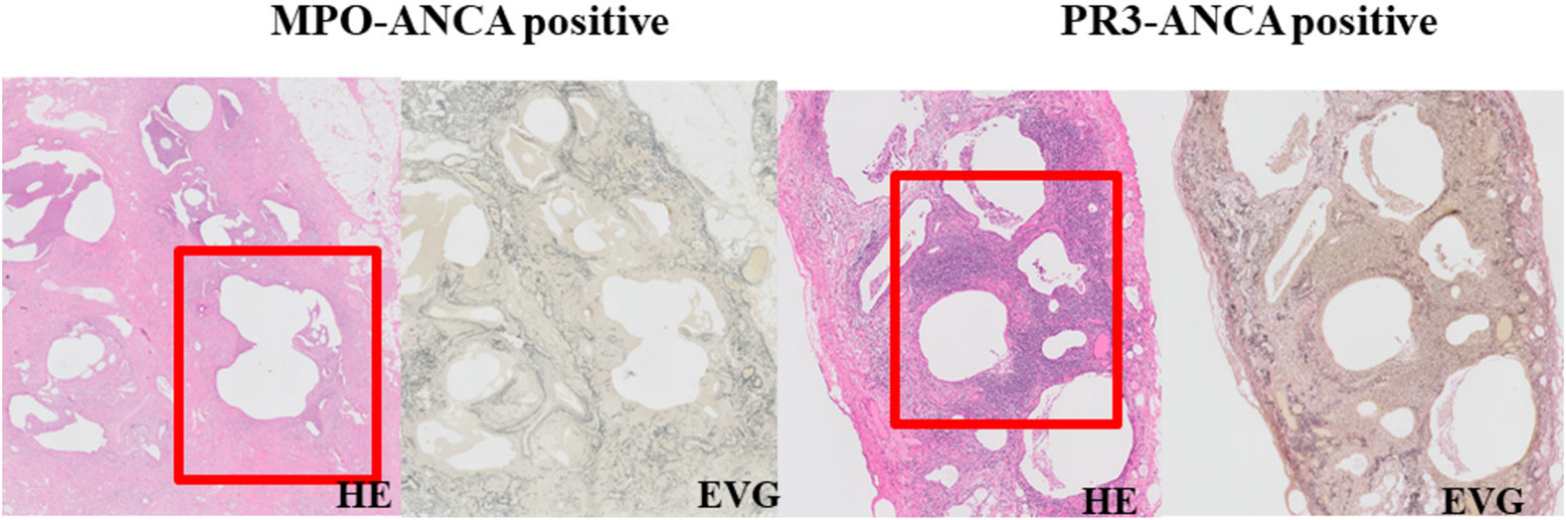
Pathological findings of increased attenuation around honeycombing on HRCT image showing lymphocytic infiltration of the bronchiolar walls inducing destructive bronchiolitis resulting cyst formation.
